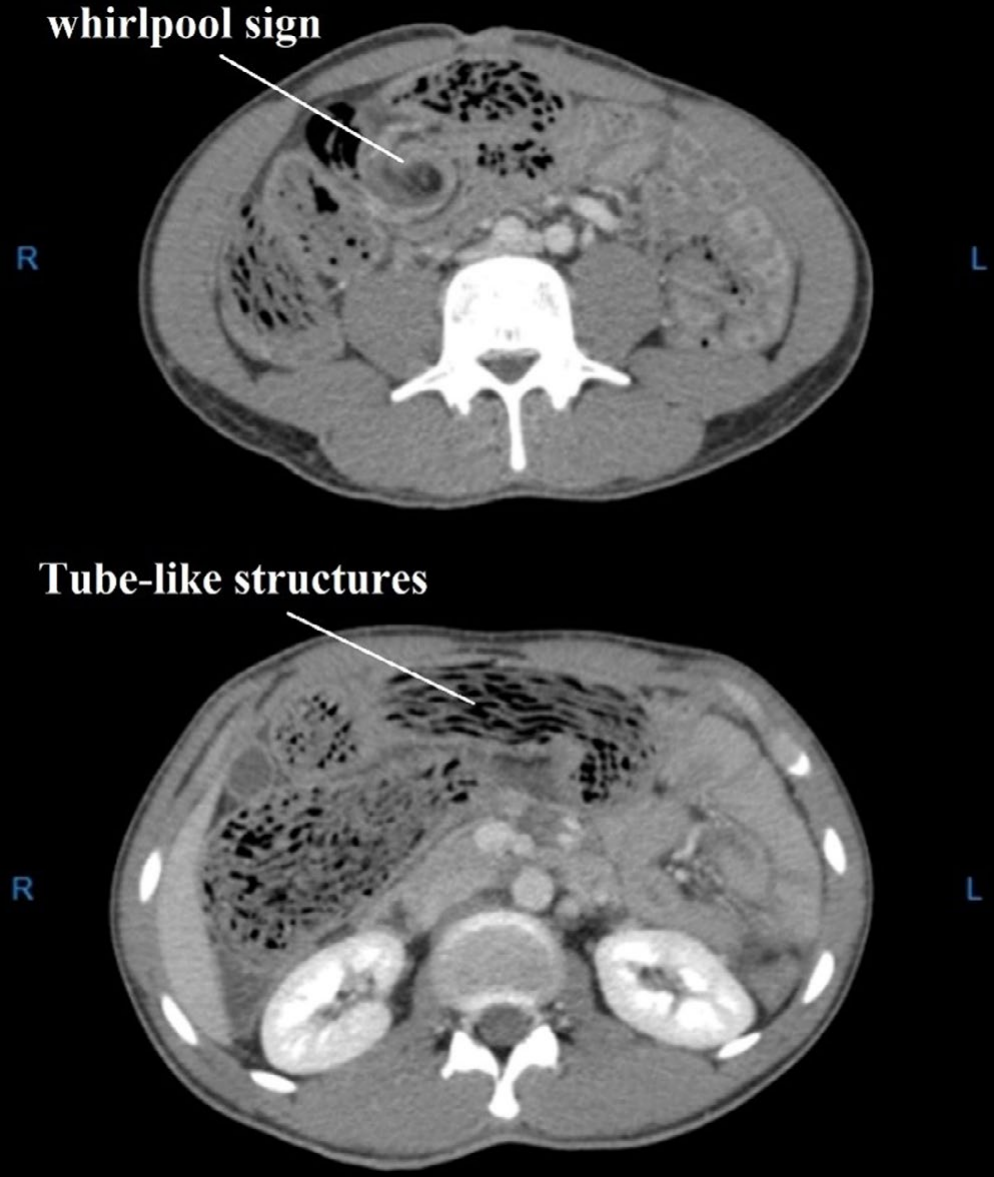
These axial CT images of the abdomen demonstrate characteristic findings of intestinal ascariasis with small bowel obstruction: “Tube‐like structures” within the intestinal lumen, which represent bundles of Ascaris worms. These appear as elongated, serpentine, non‐enhancing structures within the bowel loops. A “whirlpool sign” is observed in one section, indicative of a twisting of bowel loops, suggesting volvulus or impending obstruction due to the large worm burden. The findings are highly suggestive of intestinal ascariasis causing mechanical small bowel obstruction. The presence of a whirlpool sign raises concern for possible volvulus, which requires urgent intervention.
